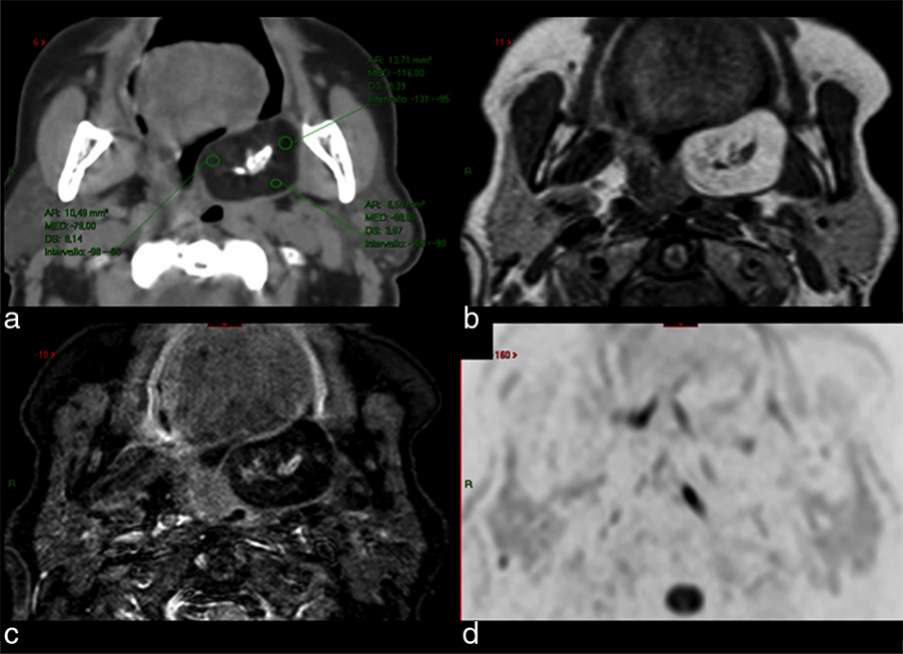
CT scan (a) and MRI [(b) axial *T*_1_ weighted; (c) axial STIR; (d) axial diffusion-weighted imaging with background suppression) show a juxtatonsillar left parapharyngeal mass with central calcification and fat tissue peripherally. Hounsfield units in the CT scan ranged between –80 and –116 (a), while fat saturation by means of STIR technique (c) confirmed the presence of fatty tissue. CT scan after ontrast media showed no enhancement (not shown). STIR, short tau inversion-recovery.
